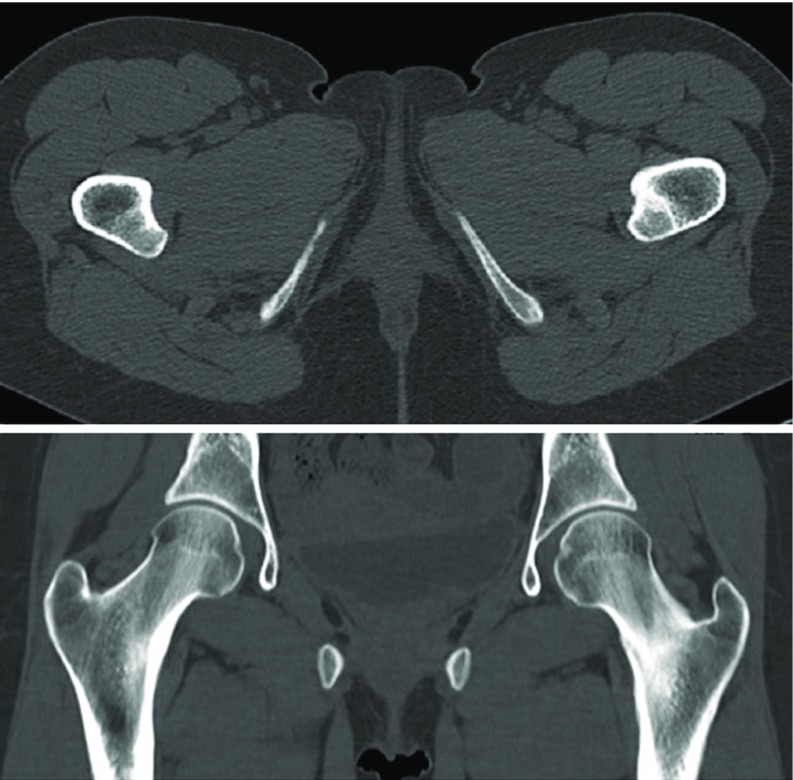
Pelvic CT-scan (A – axial view, B − coronal view) showing disruption of the medial cortex of left femoral neck.
